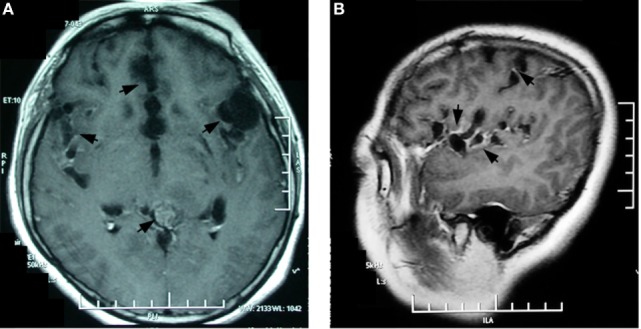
Contrasted brain magnetic resonance imaging 9 months after symptoms onset showed multiple enhanced encapsulated cyst-like lesions in the Sylvian fissure, dilated cortex sulci in the bilateral hippocampus and the medialis of bilateral frontal lobes, and a generally enhanced mass in the quadrigeminal cistern (A). The sagittal image clearly shows grape-line cysts along the Sylvian fissure (B).
